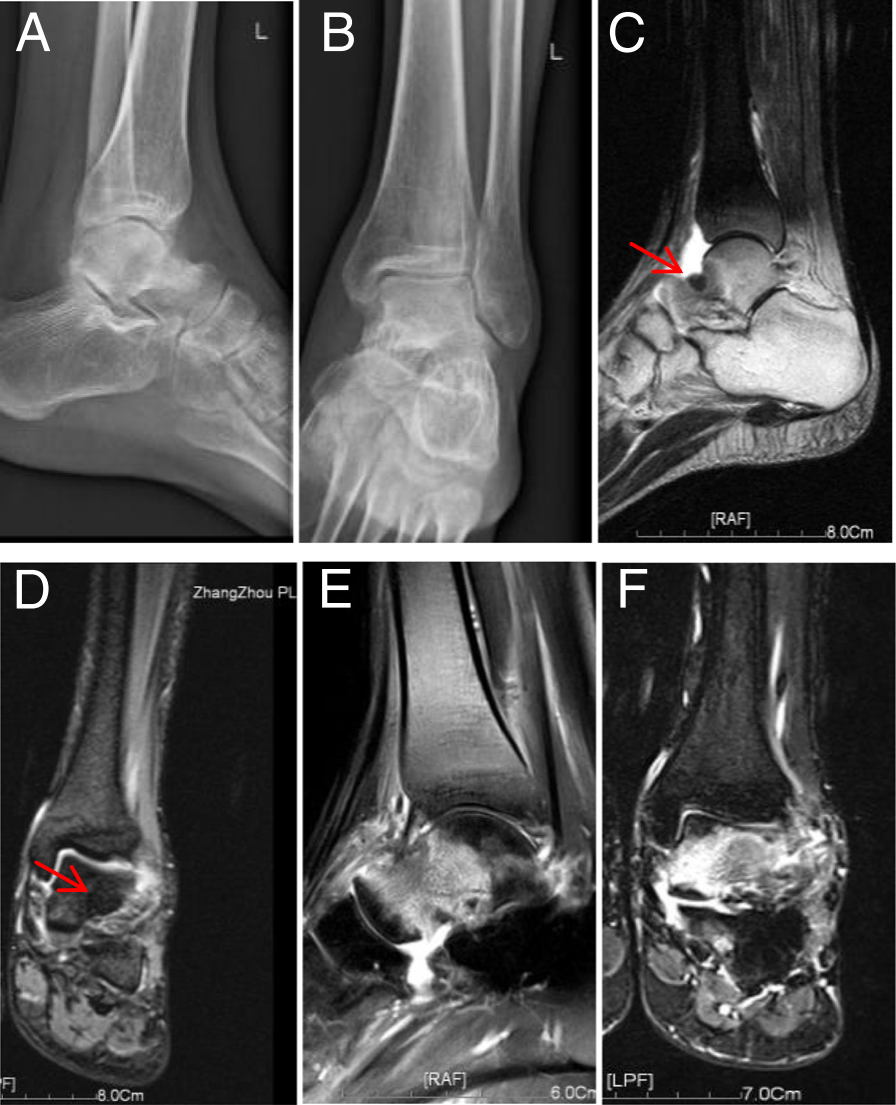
Preoperative and postoperative images at the time of the first surgery. (a, b) Preoperative lateral and anteroposterior X-rays show sclerotic lesions in the neck of the talus. (c, d) Preoperative sagittal T2-weighted and coronal T2-weighted magnetic resonance images of the left ankle show bone marrow oedema of the talus, left ankle joint effusion, and soft tissue swelling around the joint. An approximately 1-cm-diameter nodular shadow with an unclear boundary is seen in the upper front aspect of the left talus, and a small amount of effusion is present in the joint cavity (red arrow indicates the nidus). (e, f) Sagittal T2-weighted and coronal T2-weighted magnetic resonance images obtained 3 months after the first surgery show widespread bone marrow oedema of the talus
